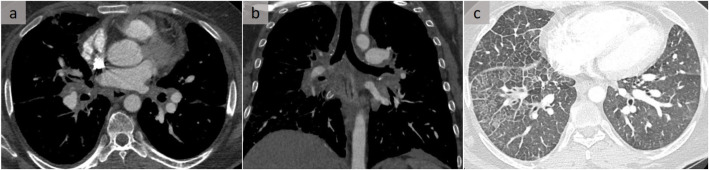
Post-tuberculous mediastinitis. A patient with chronic mediastinitis related to sequelae after tuberculosis. Axial (a) and coronal (b) CT in mediastinal windows show infiltration of the mediastinum and hila, which led to narrowing of the pulmonary veins on the right side. CT scan at the level of the heart in pulmonary windows shows right-sided septal-line thickening linked to impaired venous return (thanks to Prof. Halimi, Georges Pompidou Hospital, France)
